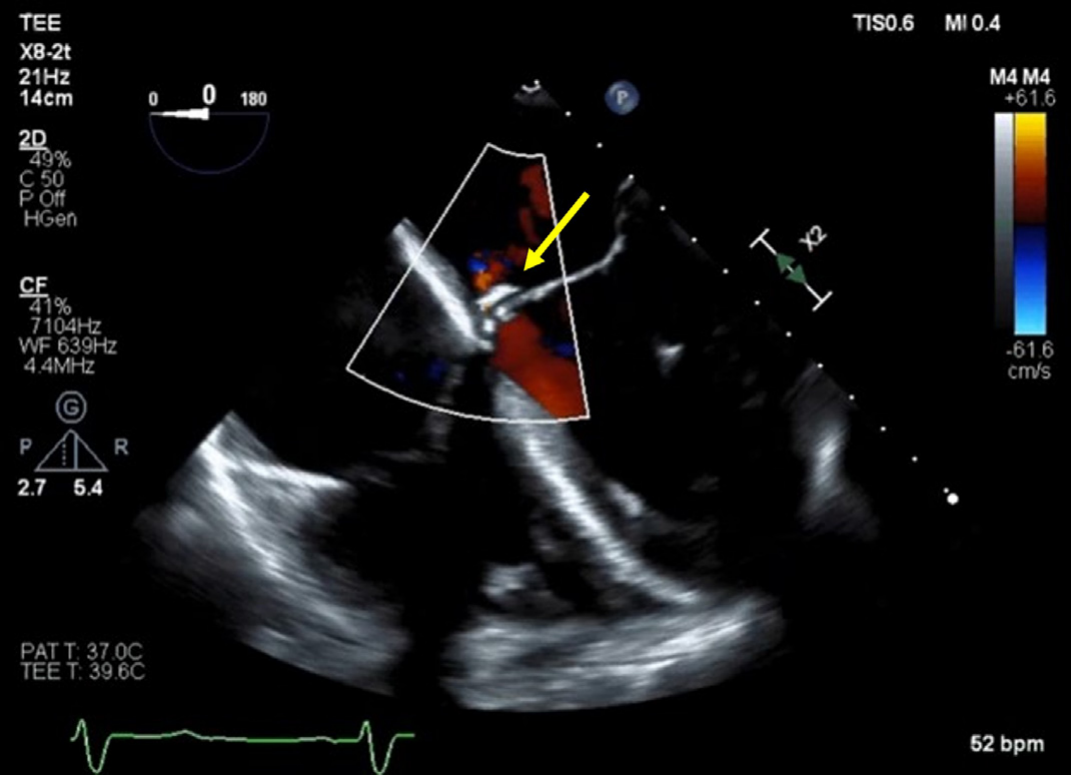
Case 1 Postprocedural transesophageal echocardiography (TEE) showed significant improvement of MR in the perforated area (yellow arrow, Amplatzer Duct Occluder II plug).
